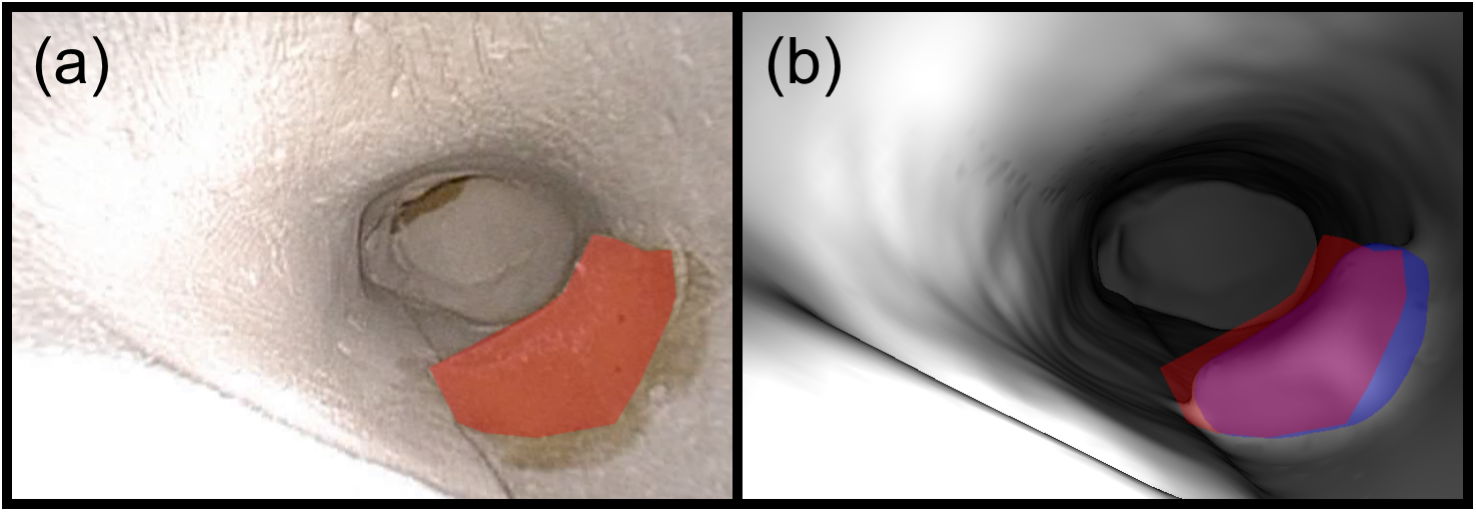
The measurement of the CT-space bolus surface. (a) A video frame showing the bolus material with the manually-drawn contour overlaid in red. The contour contains over 28,000 pixels, but downsampling reduced this to 613 pixels spaced evenly within the area. (b) The corresponding virtual endoscopic image rendered at the registered coordinates. The manually-drawn contour is overlaid in red and the image-plane projection of the bolus volume is overlaid in blue. The MAD for this frame was 0.23 cm and the Dice coefficient between the 2D contours was 0.82.
